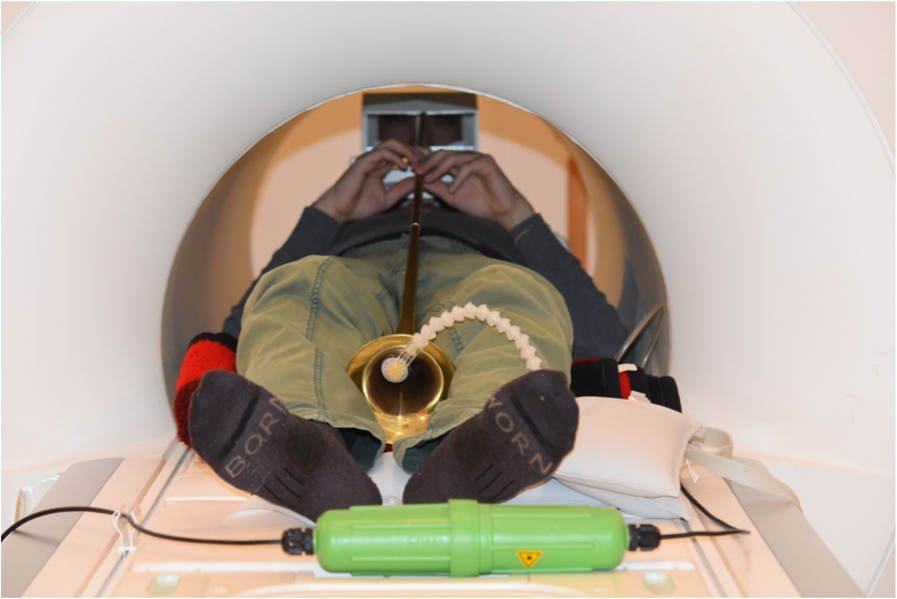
Setup for playing an MRI-compatible trumpet inside a 3 Tesla MRI system
